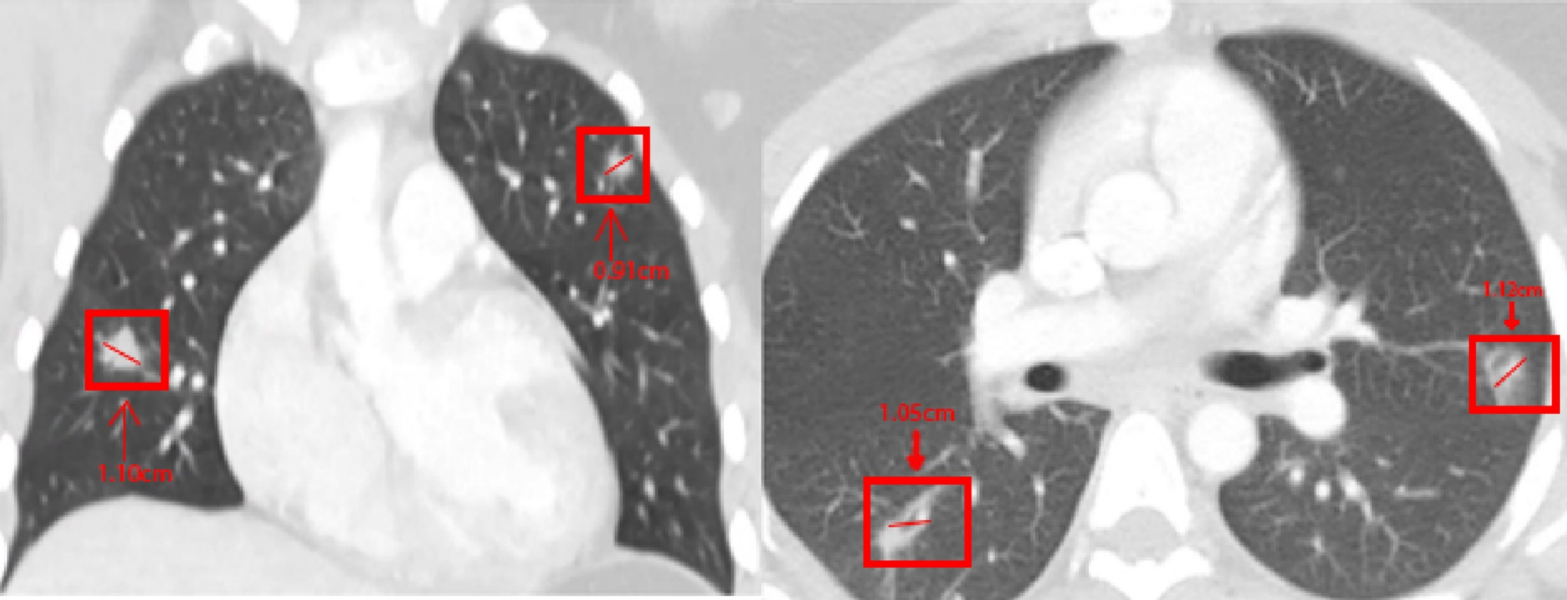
CT chest with contrast during admission with pulmonary nodules unchanged from prior CT imaging studies Left panel: axial CT chest. Right panel: coronal CT chest.
